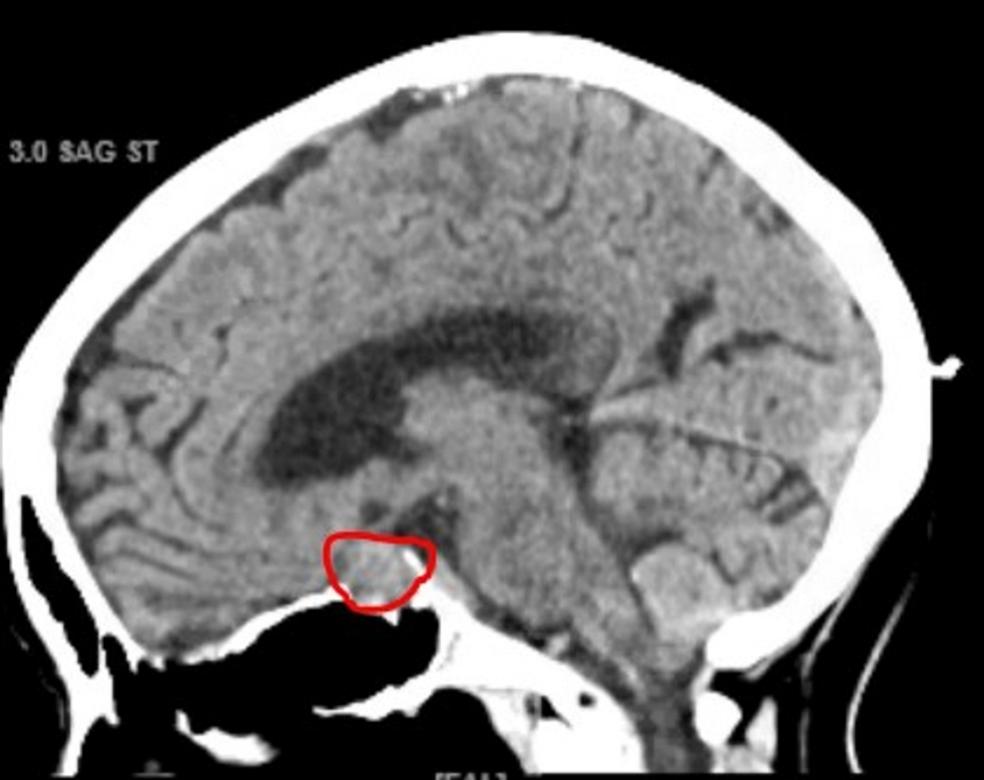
This image is a CT scan of the patient done on admission. Of note, these CT scans show a large, 15mm pituitary adenoma (red circle).
